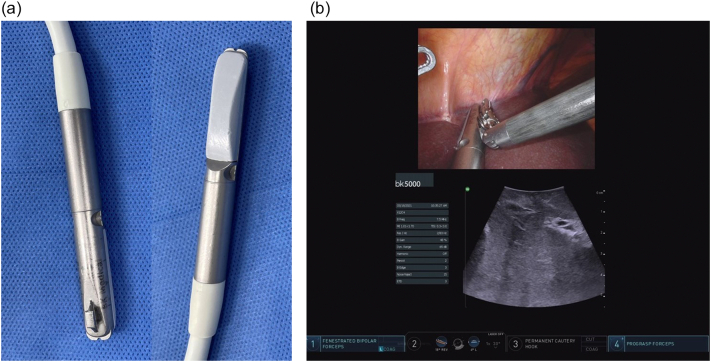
a) Curvilinear I-style probe for robotic intraoperative ultrasound, b) Surgeon's view on the robotic console enabling simultaneous visualization of both the robotic camera and ultrasound image.
